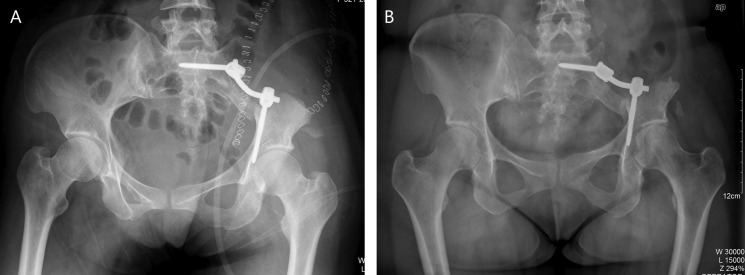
A 32-year-old woman (case 27) with diagnosis of pelvis chondrosarcoma affecting zone I (A) AP radiograph of the pelvis after internal hemipelvectomy and subsequent pelvic ring reconstruction with screw-rod system and autograft bone. (B) AP radiograph showing the bone union 12.3 months post-operatively.
